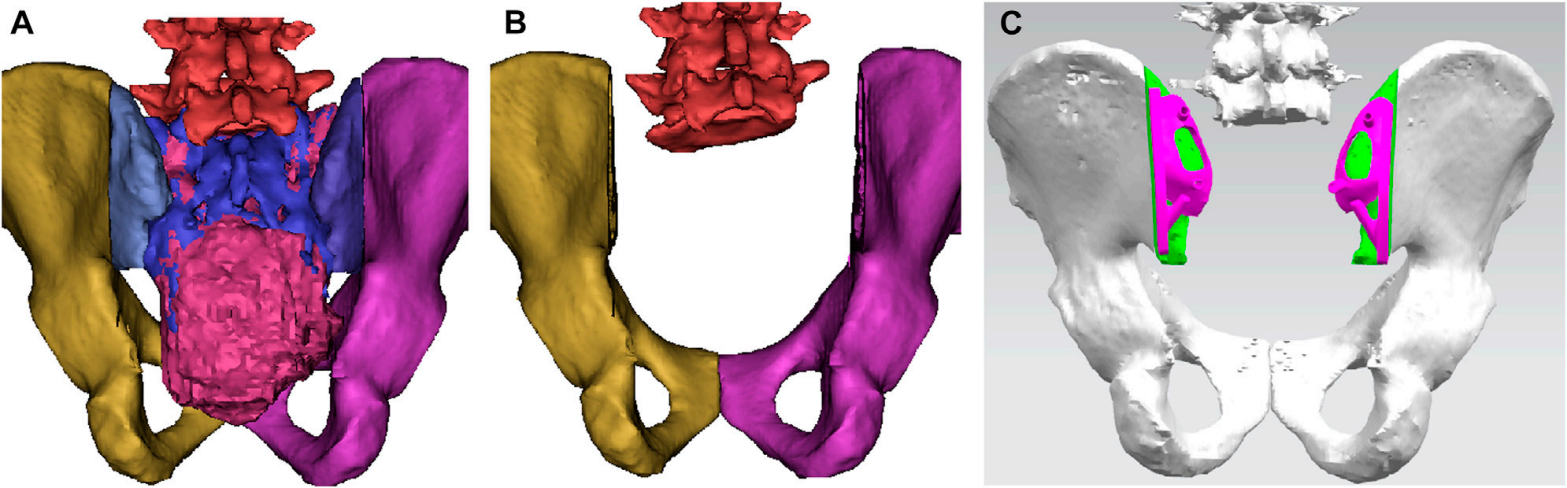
A 3D bone tumor model from CT data was created for surgical planning (A). Bone defect model after tumor resection (B). Design of the cutting guide (C).
